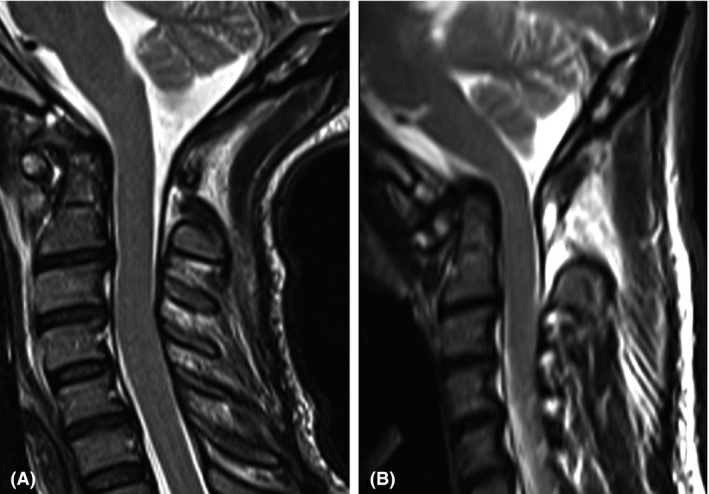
T2‐weighted sagittal MRI scans. In the early phase of the disease, only the luxation of C1–C2 seems relevant (A). The follow‐up MRI reveals basilar impression (B).
